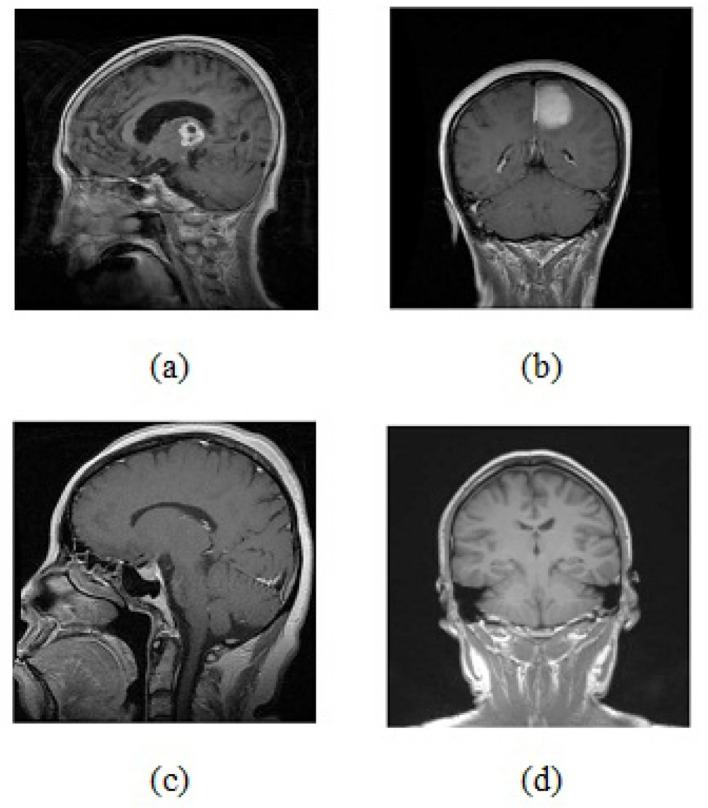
MRI images of BT: (a) glioma, (b) meningioma, (c) pituitary, (d) no tumor.
